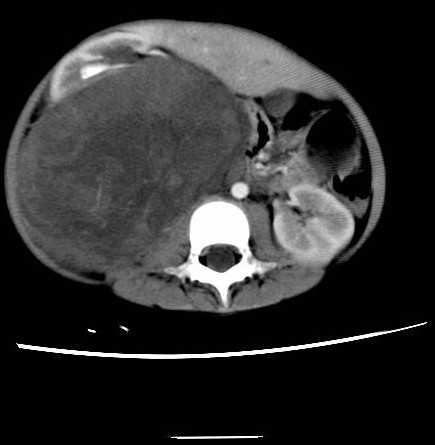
CT scan image of the tumor.
